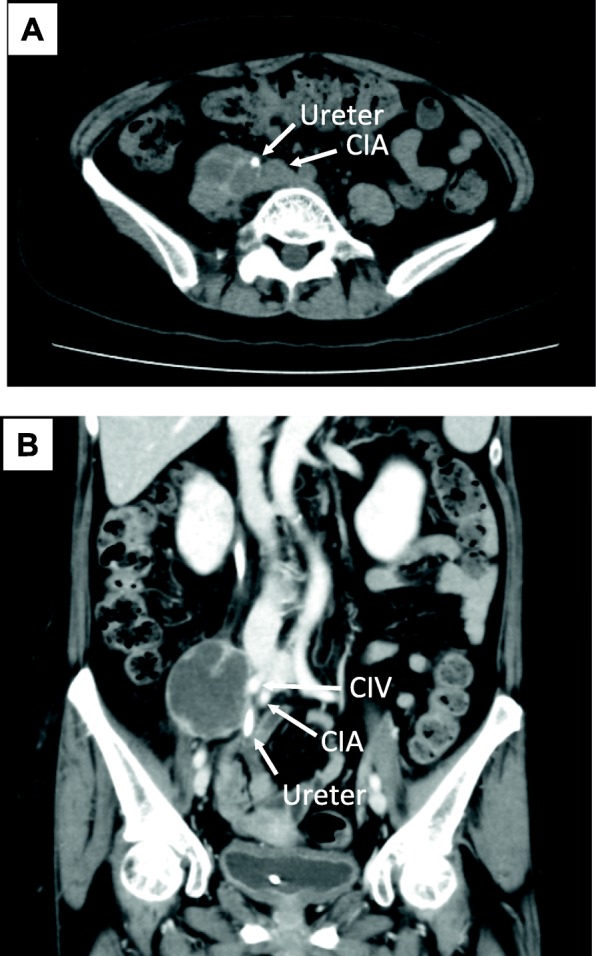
CT scans of retroperitoneal tumors after 6 courses of chemotherapy with AI. a Axial image. b Sagittal image after multiplanar reconstruction. CIA, common iliac artery; CIV, common iliac vein
